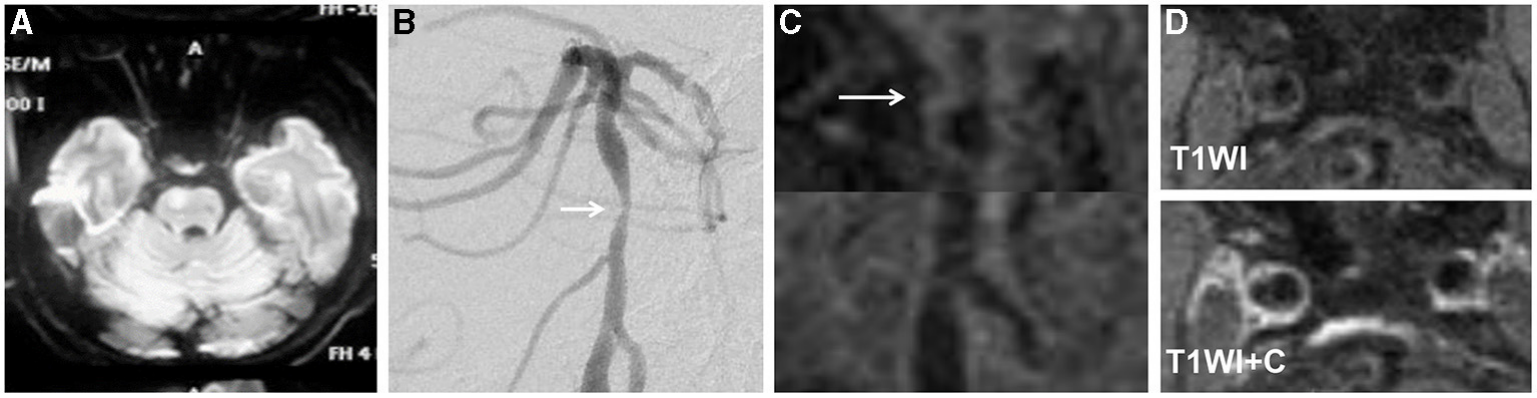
A 68-year-old man presented with dysarthria for 20 days. The DWI showed an infarct located at the right pons (A). The DSA showed severe stenosis in the middle segment of the basilar artery (arrow) (B). The HRMRI demonstrated a diffuse distributive plaque (arrow) (C). There was no plaque enhancement on T1-weighted image after gadolinium–DTPA injection (D).
